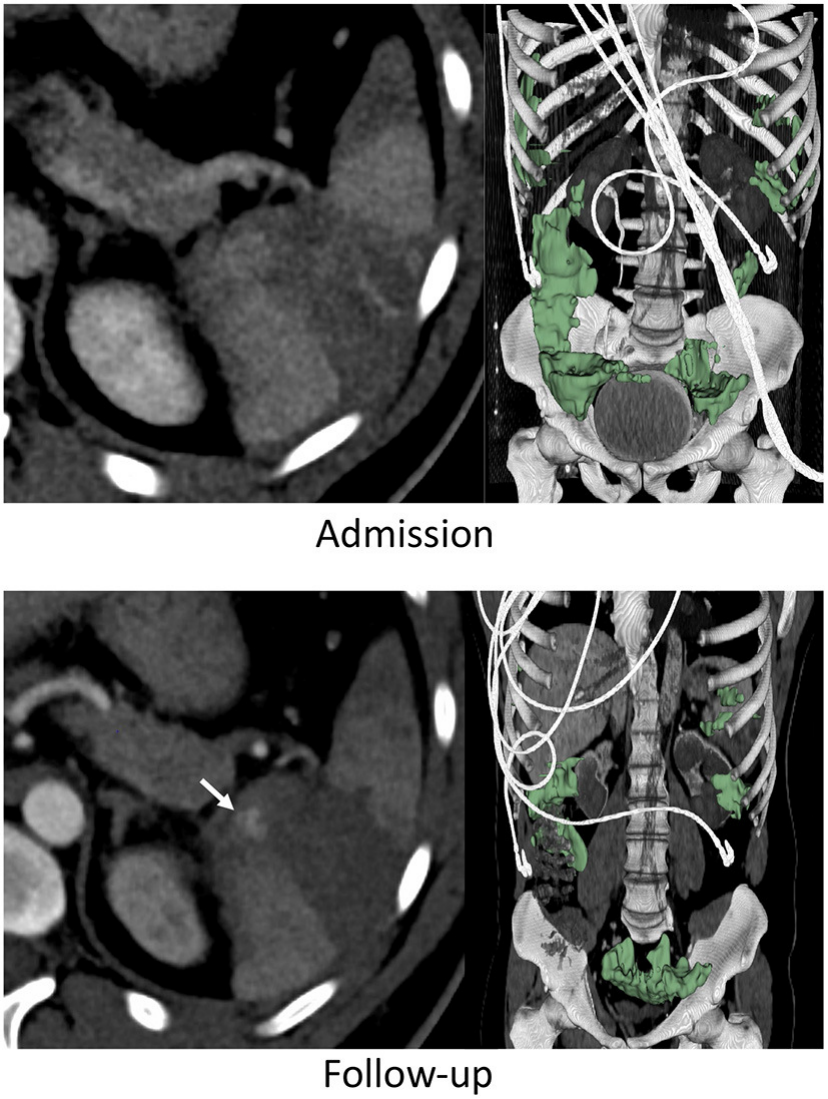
Thirty three years-old male with BSI successfully managed non-operatively with adjunct AE. Admission CT shows an AAST grade 3 injury. A new pseudoaneurysm was seen on follow-up performed 25 h later (arrow) but hemoperitoneum volume (green label mask) had decreased from to 262 to 222 mL.
